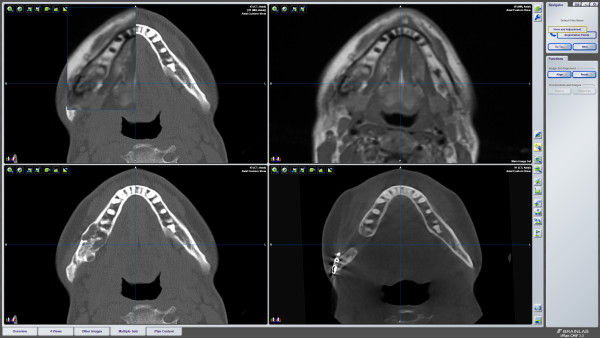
Preoperative CT and MRI scans showing the heterogeneous lesion in the right mandible with no vascular signs.
